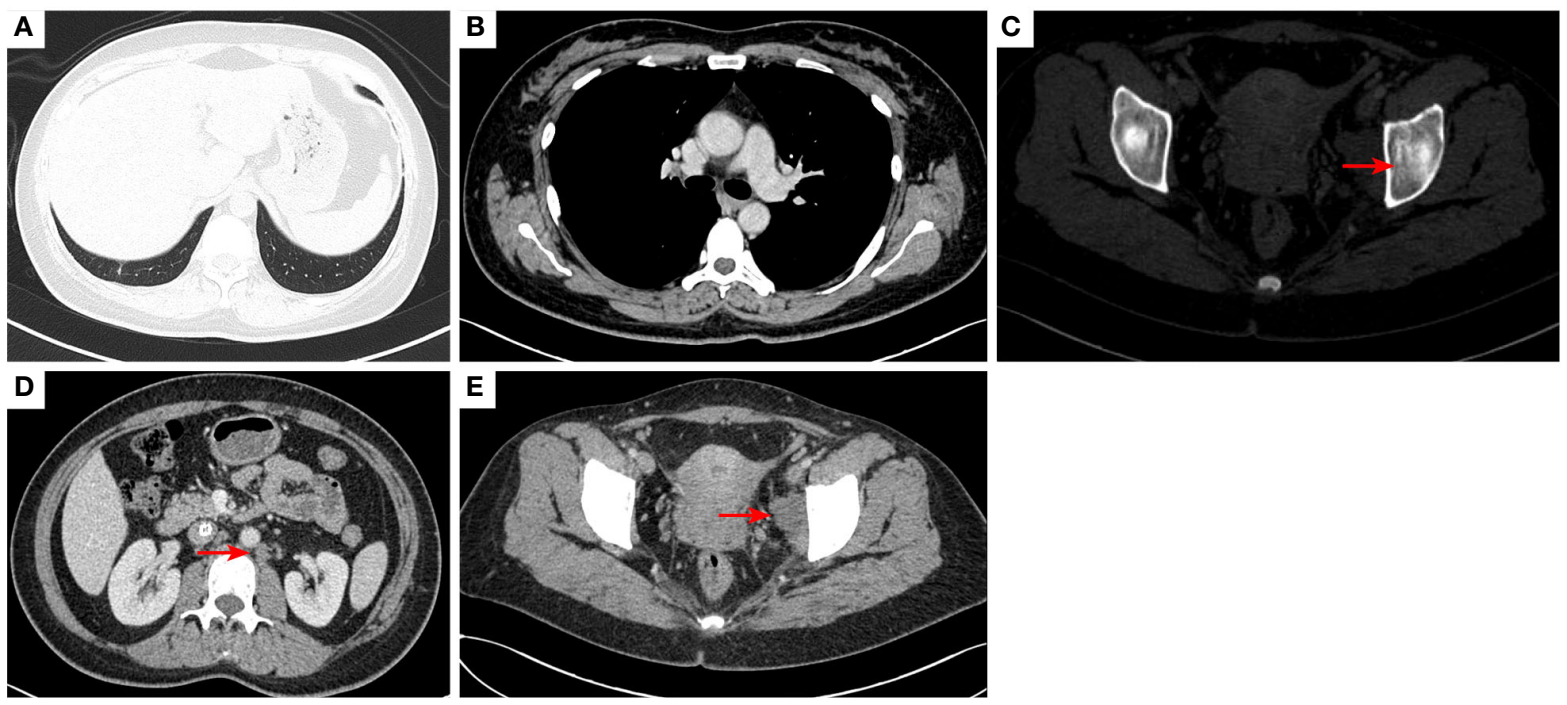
2020–9-16 CT scan: The original metastatic tumor in the right lower lobe of the lung disappeared (A); no enlarged lymph nodes were found in the right hilum (B). Left acetabular metastases [red arrow, (C)]. The short diameter of retroperitoneal lymph nodes was 0.91 cm which was a non-confirmed metastases [red arrow, (D)], and the short diameter of left pelvic lymph nodes was 2.98 cm [red arrow, (E)]. The efficacy was SD. SD, stable disease.
